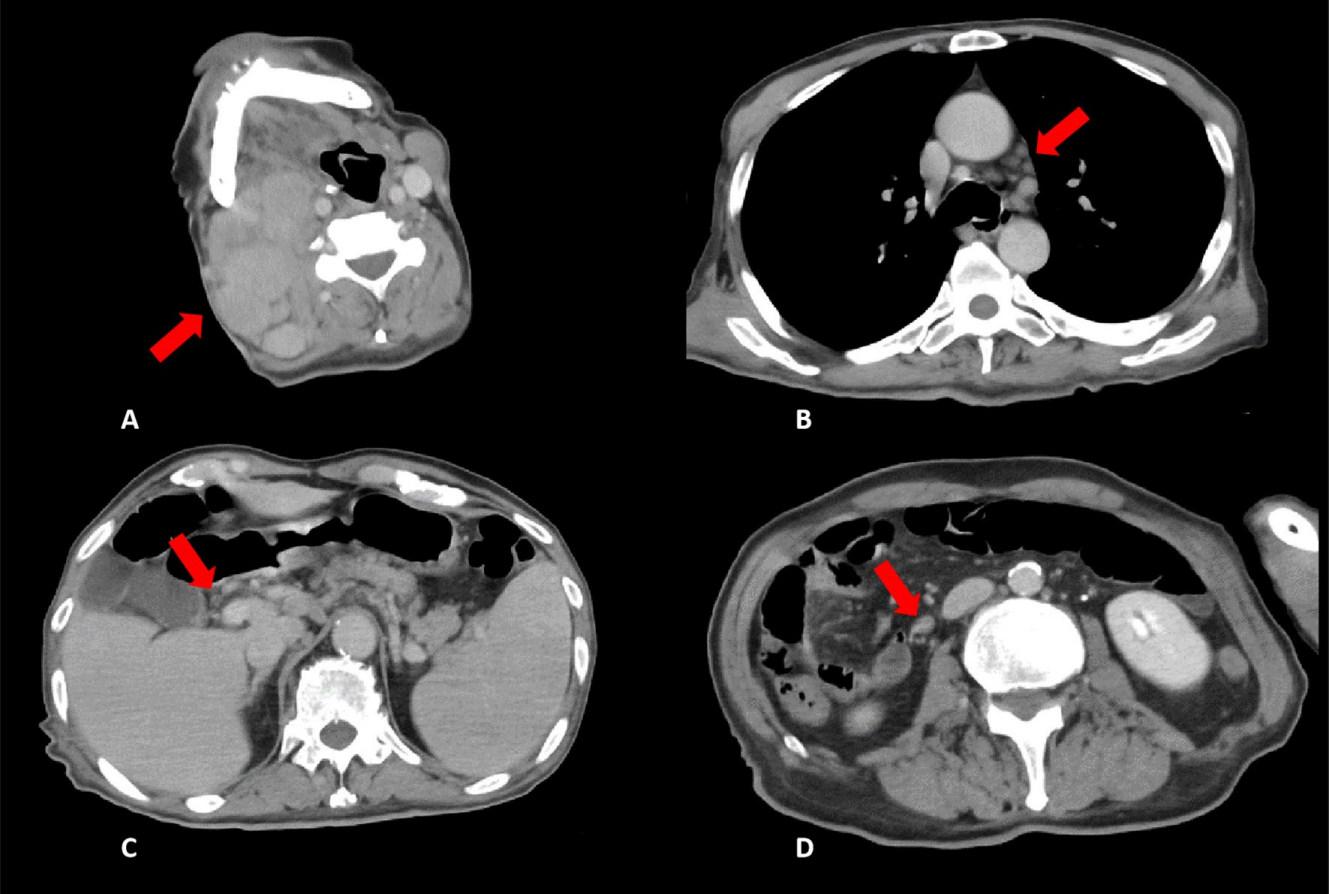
Contrast-enhanced computed tomography (CT) images demonstrated swelling of multiple lymph nodes. Red arrows indicate A right cervical lymph nodes, B mediastinal lymph nodes, and D intraperitoneal lymph nodes. C Mild dilatation of the extrahepatic bile duct with contrast enhancement and splenomegaly
